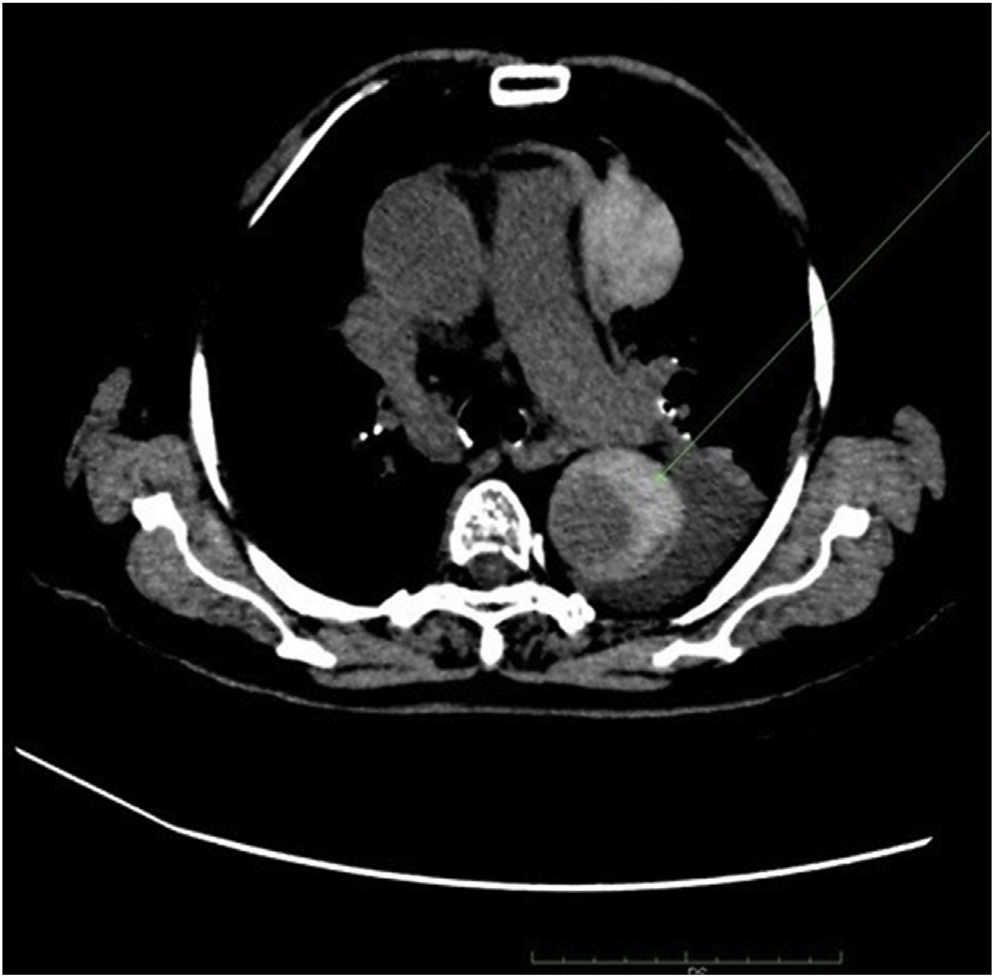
Hyperdense crescent-like aortic wall hematoma on computed tomography.
